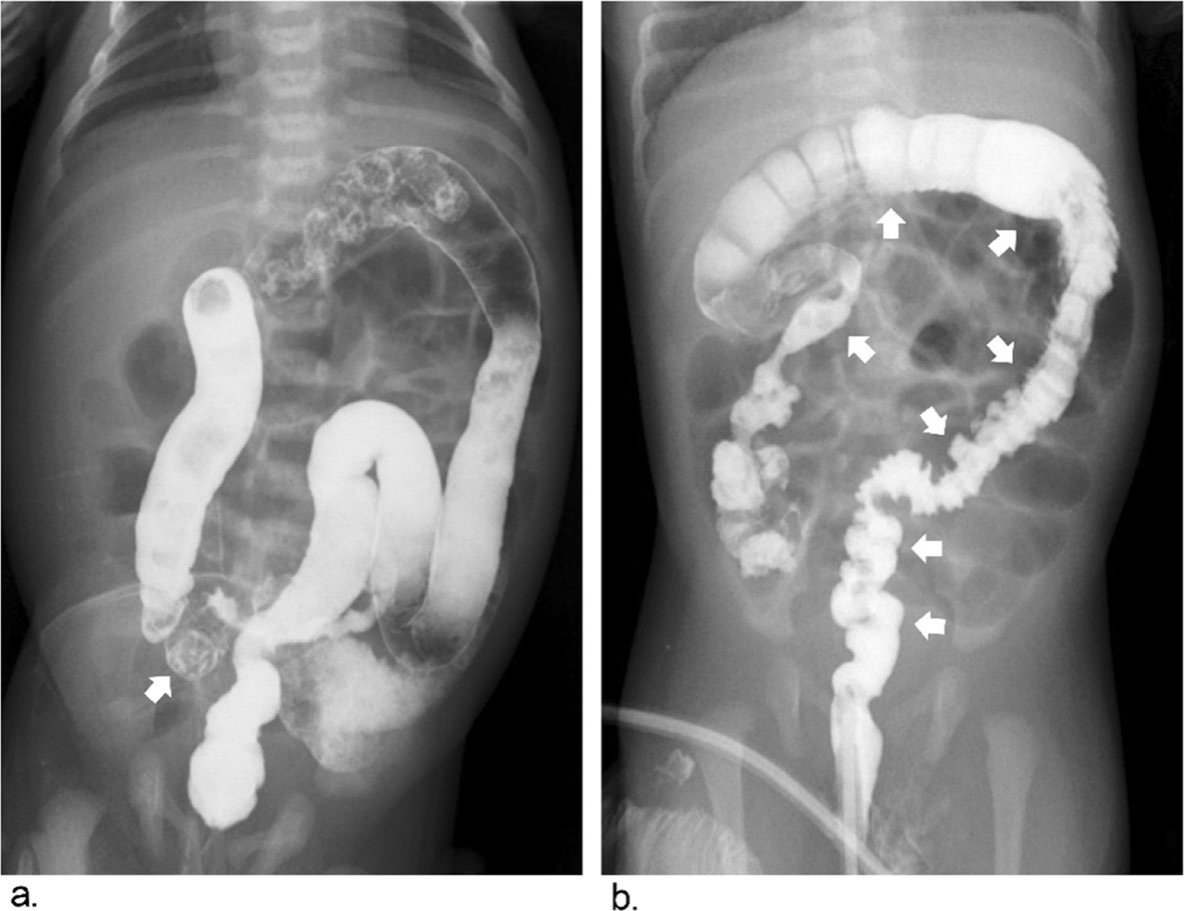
Images show common and typical radiographic signs of preoperative BEs in patients with TCA. a Ileocecal valve reflux, reflux of contrast material into the terminal ileum *(arrow)*. b Question-mark-shape colon, described as a rounded and shortened contour of hepatic and splenic flexures with short appearing colon *(arrows)*
